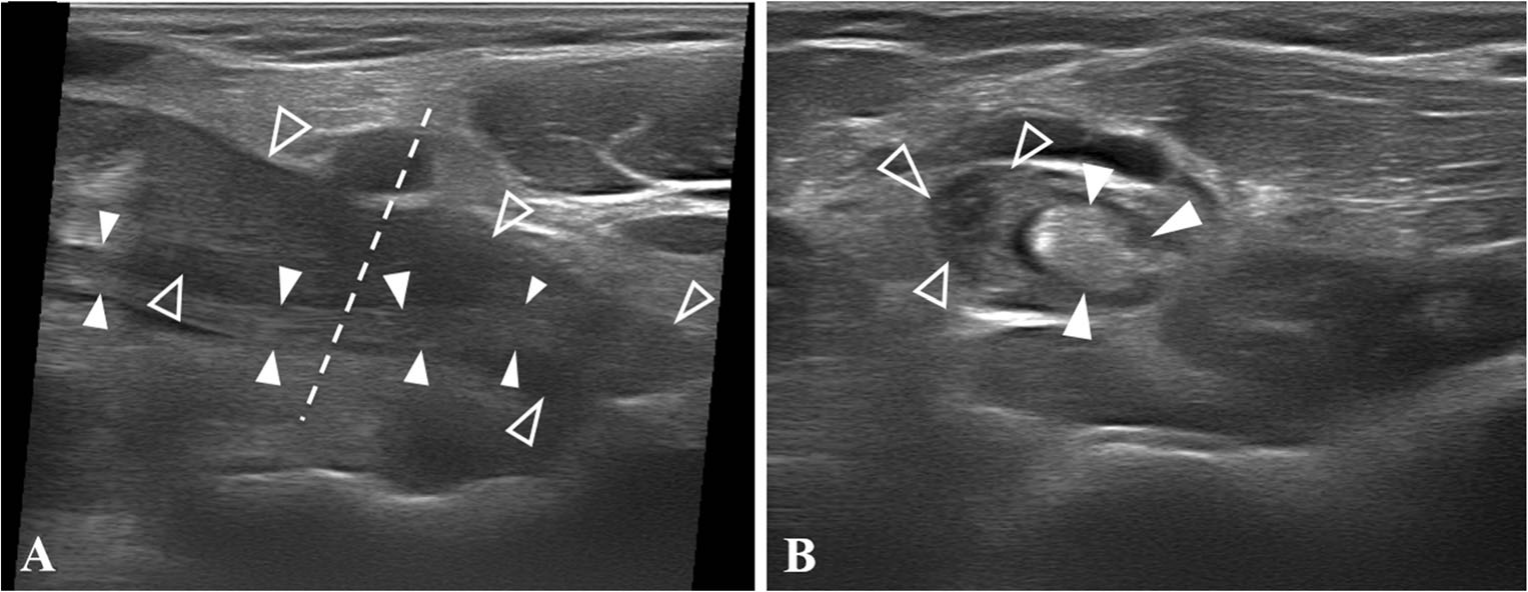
Ultrasound view of a type 1A injury (elongation or traumatic injury without evidence of tendon-tearing affecting mainly one of the two DBBT components). a In the long-axis view, different echotextures and morphologies are observed in the elongated long-head component (*empty arrowheads*), i.e. hypertrophic and hypoechogenic, and the normal short-head component (*white arrowheads*), i.e. normal thickness and fibrillary pattern. As the DBBT rotates distally, both components cross over each other on the longitudinal axis. The *dashed line* shows the point where image b was obtained. b Short-axis view of a. The same findings are observed in a cross-section view. An anechoic interface between the two DBBT components is observed. We have considered it a detachment of both components at the level of the endotenon septum owing to shear stress when the long-head component was elongated
